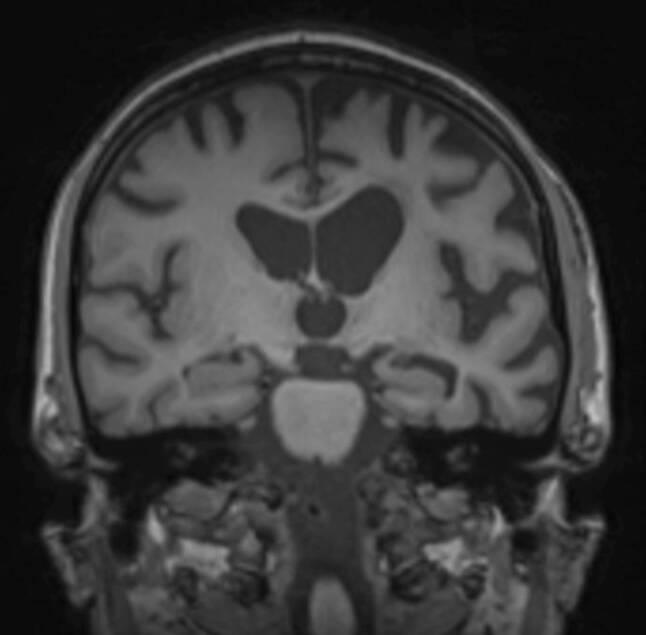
Frontotemporal dementia. A 60-year-old male patient with frontotemporal dementia, who presented with asymmetric frontotemporal atrophy depicted on coronal T1-weighted images
